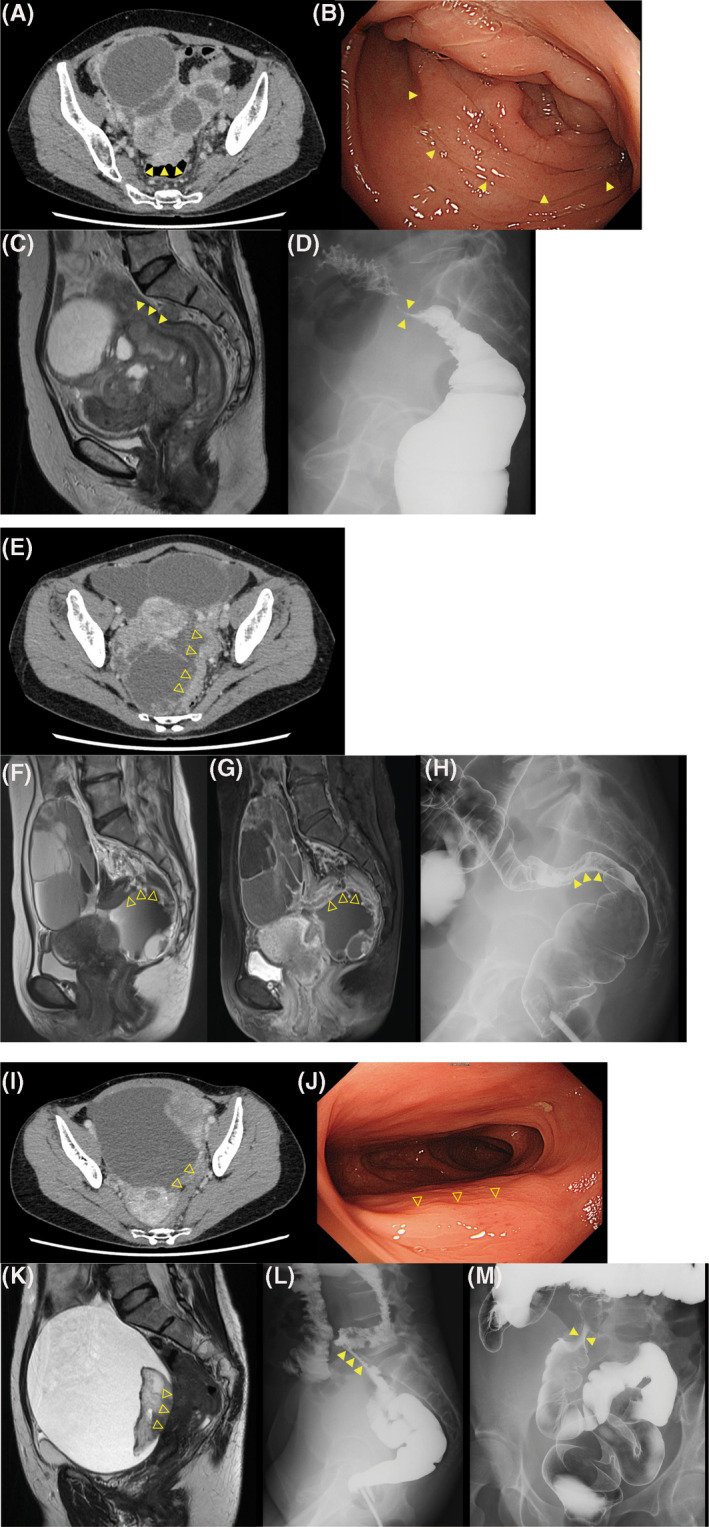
(a–d) Preoperative imaging evaluation of case 1. Patient with ovarian serous carcinoma that needed colorectal resection and pathologically proven to have invasion. In this patient, computed tomography (CT), magnetic resonance imaging (MRI), barium contrast radiography, and colonoscopy all showed invasive findings. (a) Enhanced CT, which indicated a rectal invasion by thickening of the rectum wall (arrowhead). (b) Colonoscopy revealed thickened and edematous mucosa of the rectum with stenosis indicating invasion of carcinoma. (c) MRI showed the interruption of the rectal wall. (d) Barium contrast radiography showed severe stenosis with extension in the anal side of the rectum. (e–h) Preoperative imaging evaluation of case 2. Patient with ovarian serous carcinoma that needed colorectal resection and pathologically proven to have invasion. In this patient, only barium contrast radiography showed positive finding of invasion e, CT showed that rectum came close to the ovarian tumor; the invasiveness of the cancer is not clear. (f) (T2‐weighted) and G (enhanced T1‐weighted) MRI, it was difficult to detect direct invasion to other organs (arrowheads). (h) Barium contrast radiography showed extension from outside of the rectum. Insufflation revealed strong twitching of mucosa, which indicates the intestinal invasion. (i–m) Preoperative imaging evaluation of case 3. Patient with ovarian clear cell carcinoma and operatively free of intestinal invasion but positive for barium contrast radiography (false positive). (i) CT showed retraction of sigmoid colon with some space between the tumor (arrowheads). (j) Colonoscopy showed extra‐intestinal oppression with no evidence of invasion (arrowheads). (k) MRI showed a large ovarian tumor. However, there was no invasive sign to the rectosigmoid colon. l (side view) and m, (front view), barium contrast radiography indicated the rectosigmoid invasion by strong stenosis (arrowheads)
